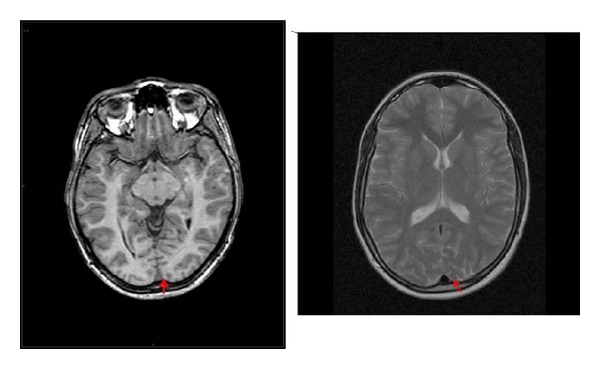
MRI scan of the brain and orbit. The ventricles appear normal. The white matter thickness around the cerebral hemispheres is normal but the grey matter (as shown by red arrows) around the occipital poles is diminished in thickness and poorly defined. The brain folding and myelination are normal.
